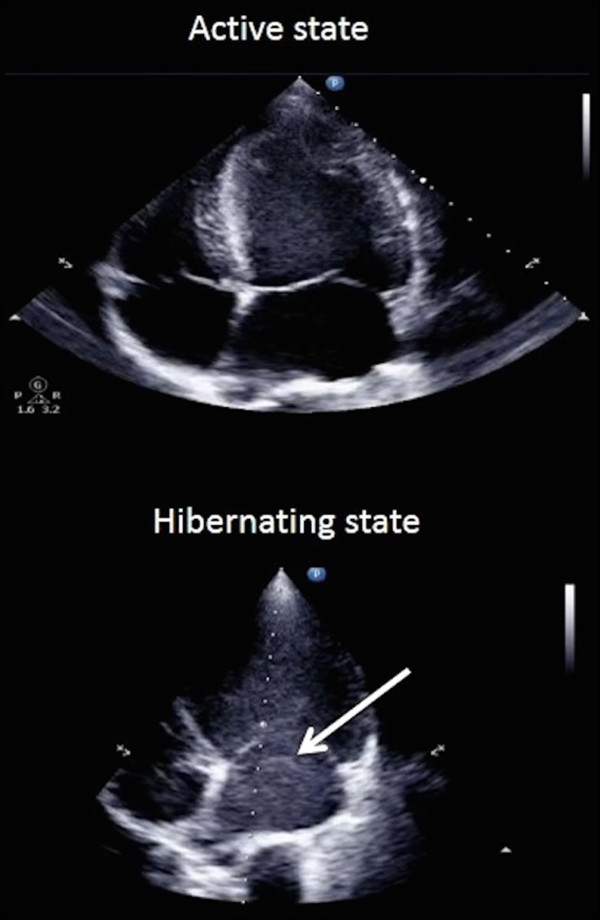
Comparison of 2D echocardiographic images from the hibernating and active states of free-ranging brown bears. An arrow indicates the presence of spontaneous echo contrast as echo-dense shadows during hibernation. Also see Additional files 1 and 2.
